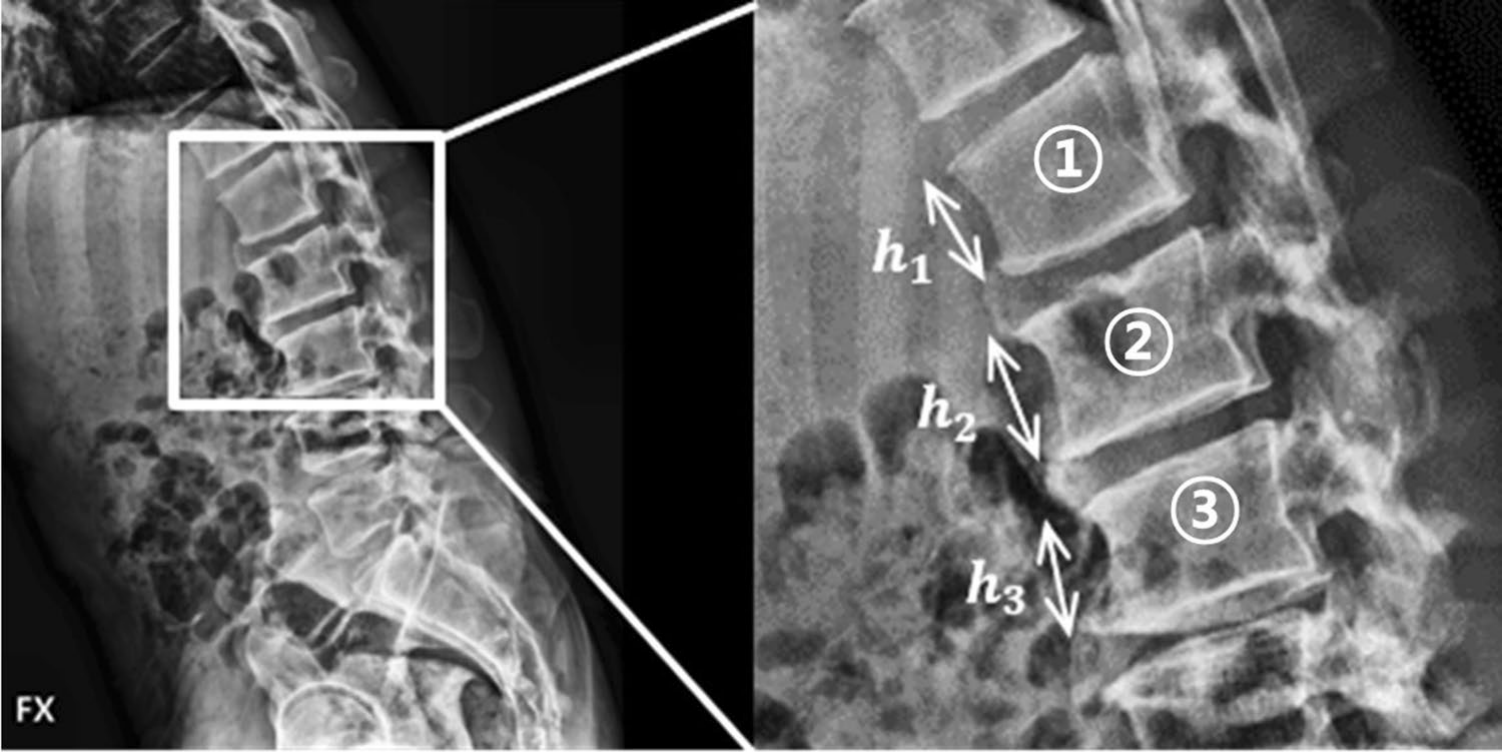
Vertebral X-ray image used for the training, and the anterior vertebral height used for calculating the vertebral compression.
